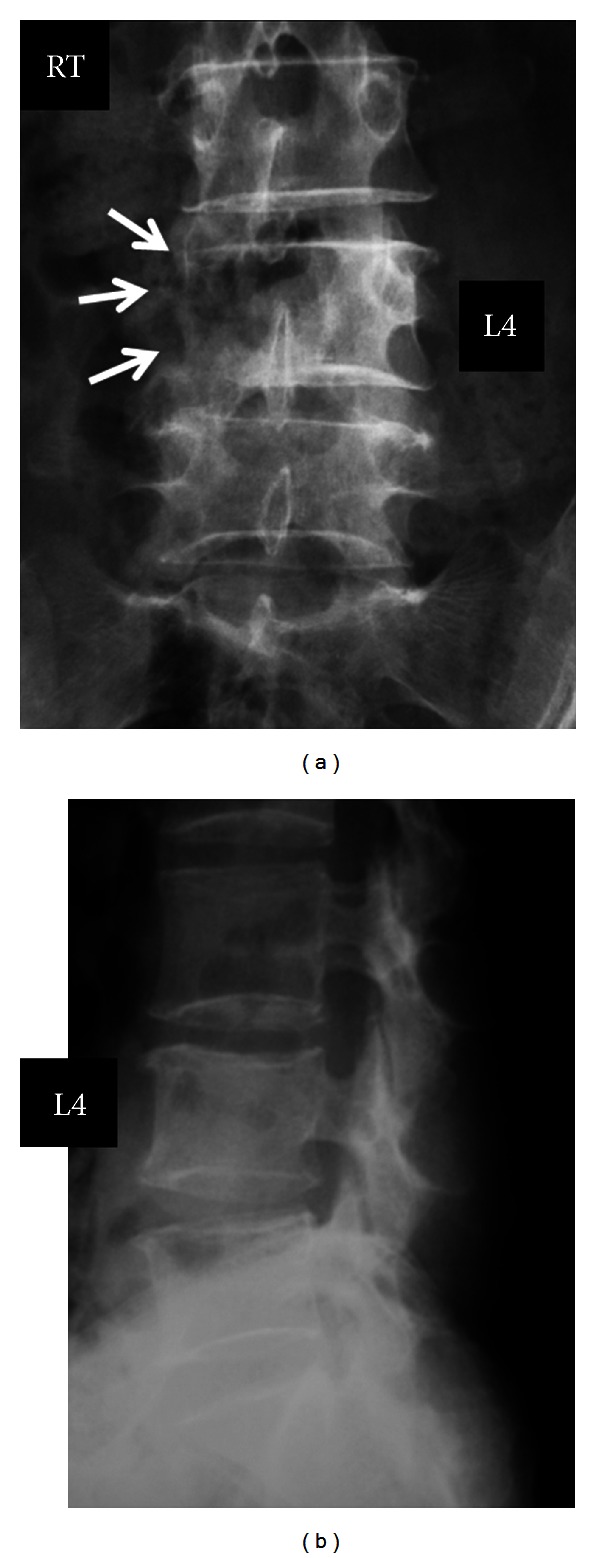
Plain radiographs (June 2006) at first consultation clearly show bone destruction of the right L4 pedicle as the winking owl sign, which suggests a metastatic malignant tumor of the spine.
